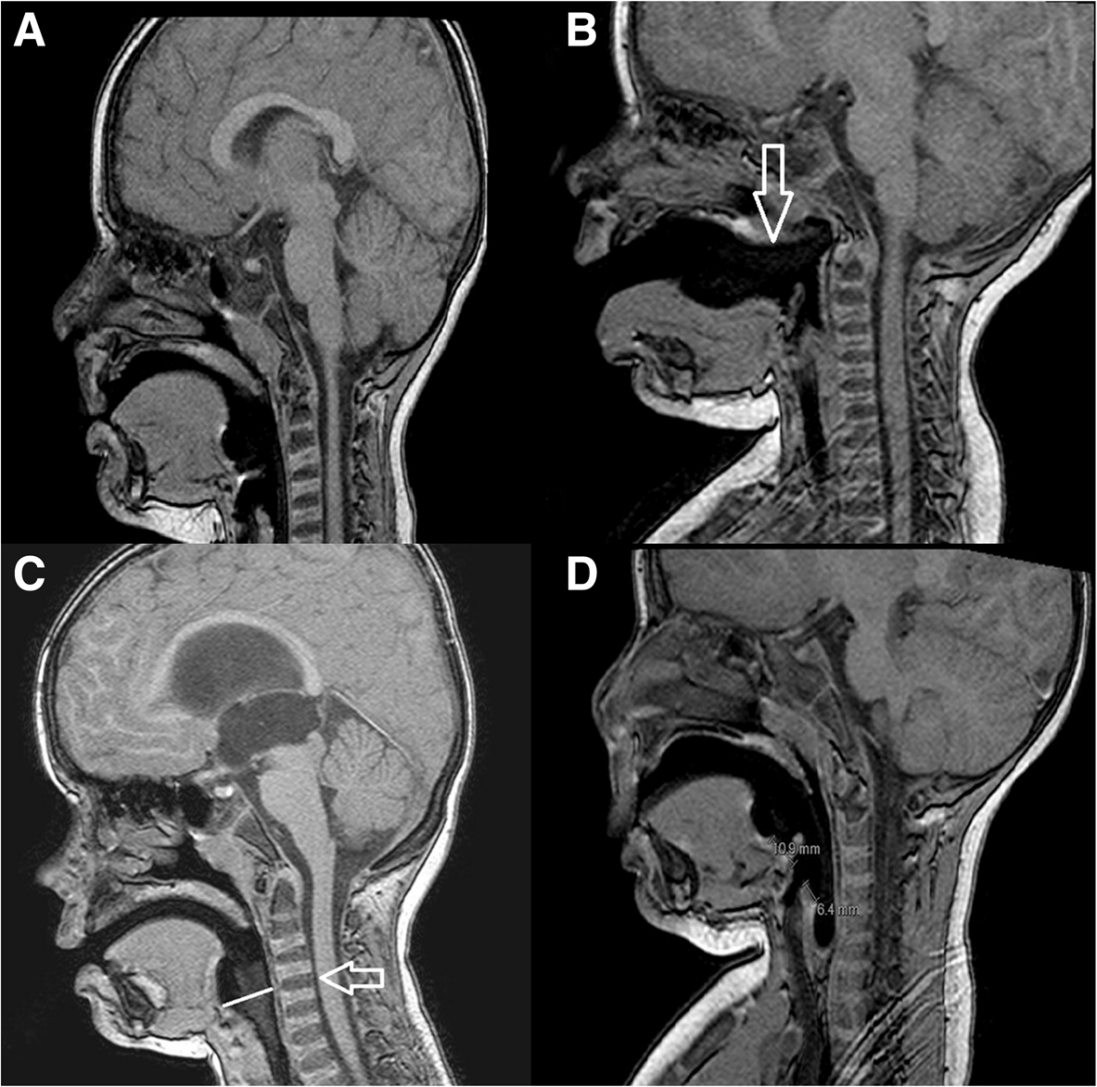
Laryngeal masks positions on MRI image. a Correct position of the LM; b Malposition A; c Malposition B; d Malposition C
